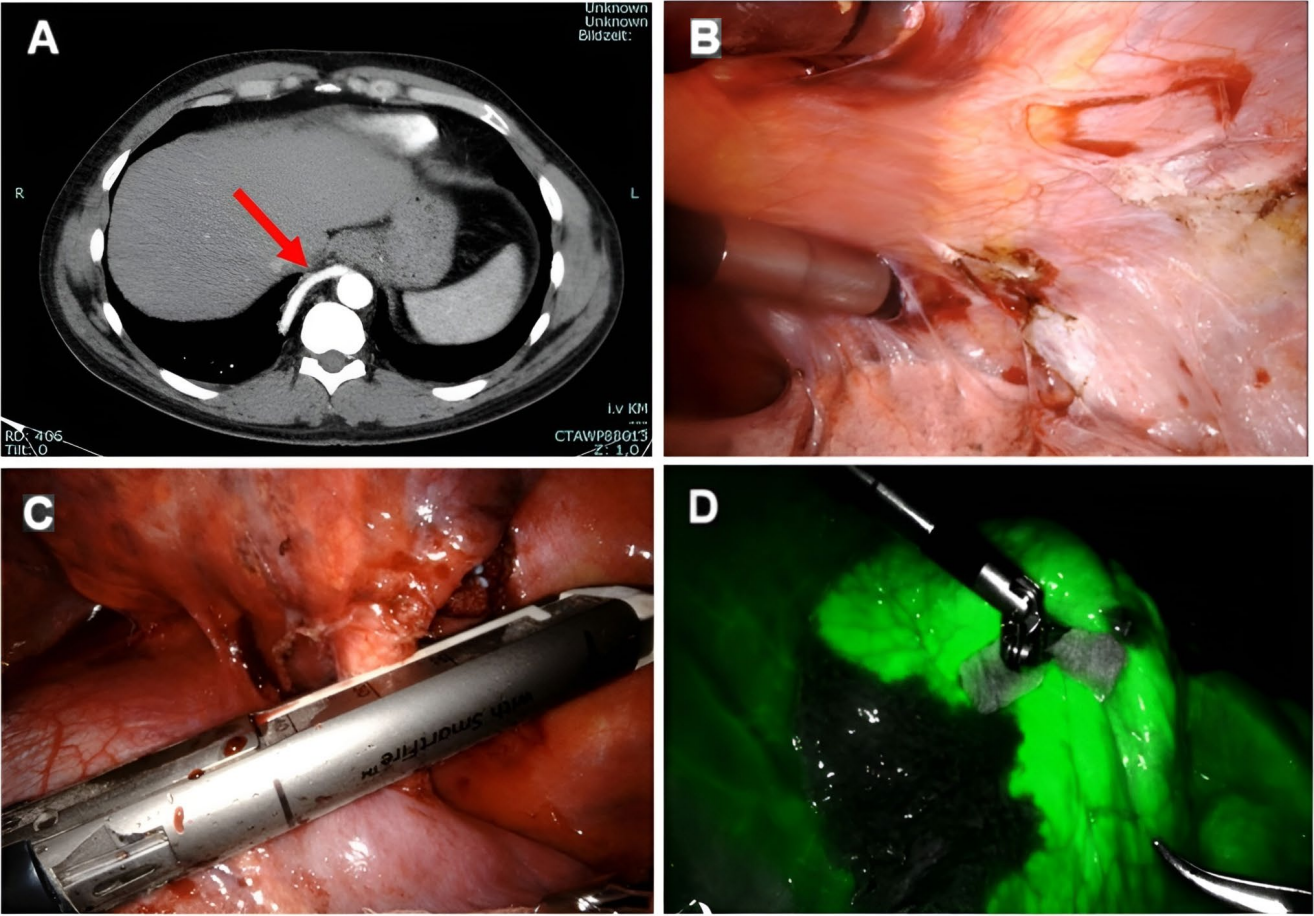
A CT scan showing a right-sided intralobar sequestration with an arterial vessel originating from the descending aorta; B Extensive adhesiolysis; C Vessel ligation using a robotic stapler; D Usage of ICG for parenchymal margin identification
